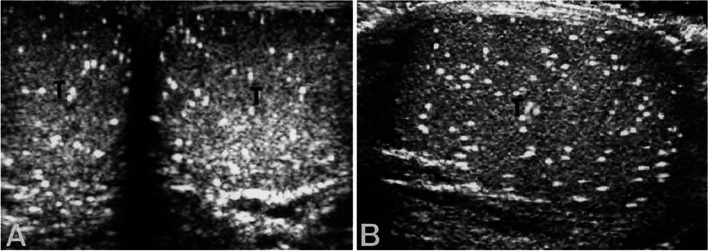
Ultrasound Scan of Classic Testicular Microlithiasis. Ultrasound Scan of Classic Testicular Microlithiasis in a 35-year-old male. A: both testes, transverse view. B: right testis, longitudinal view. Image by Kim et al. [6] used with permission
